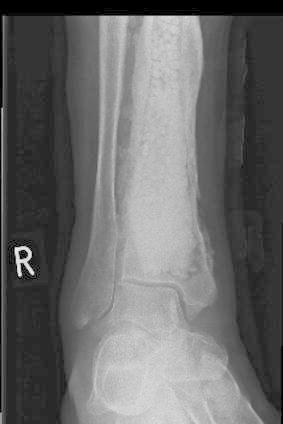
Post-operative plain radiograph of right distal tibia packed with OSTEOSET^®^-T
